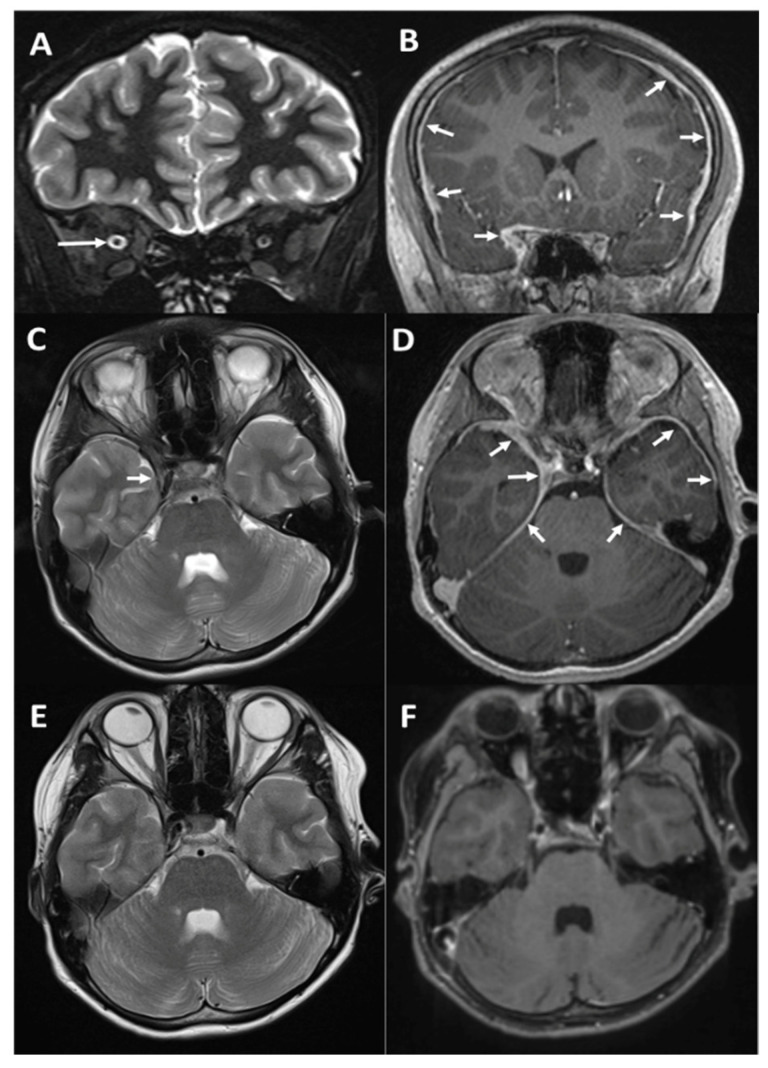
MRI findings in our patient. Top row (A,B): Coronal STIR image at presentation showing mild congestion of the right orbit with a dilated optic nerve sheath but normal signal of the optic nerve ((A), long arrow); coronal post-contrast T1w (T1 weighted) image showing diffuse dural thickening extending to the right cavernous sinus and optic canal, where it surrounds the optic nerve ((B), small arrows). Middle row (C,D): Axial T2w (C) and post-contrast T1w (D) images showing normal brain appearances but diffuse bilateral dural thickening with subtle corresponding low signal on T2. Bottom row ((E,F)): Follow-up imaging after a month showing resolution of the dural enhancement, though there is still some evidence of right 6th cranial nerve palsy (note the adducted right globe).
